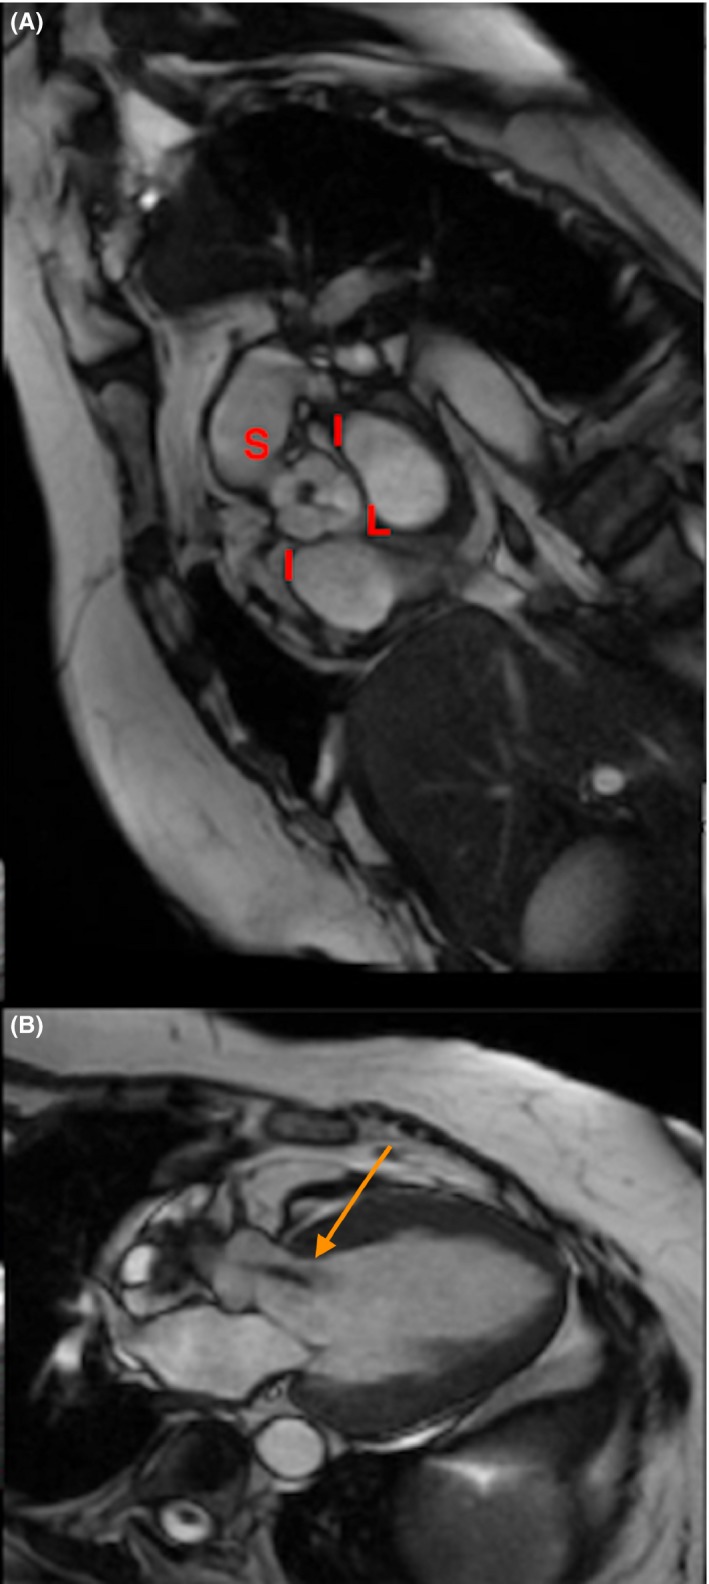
Cardiac magnetic resonance images. A, Short‐axis cine of aortic valve shows a central non‐coaptation of a quadricuspid aortic valve type D (four cusps: one large (L), one small (S), and two intermediate (I)) according to the Hurwitz & Roberts classification. B, The three chamber view in end‐diastole shows a dilated left ventricle and non‐quantifiable aortic valve regurgitation (see arrow)
